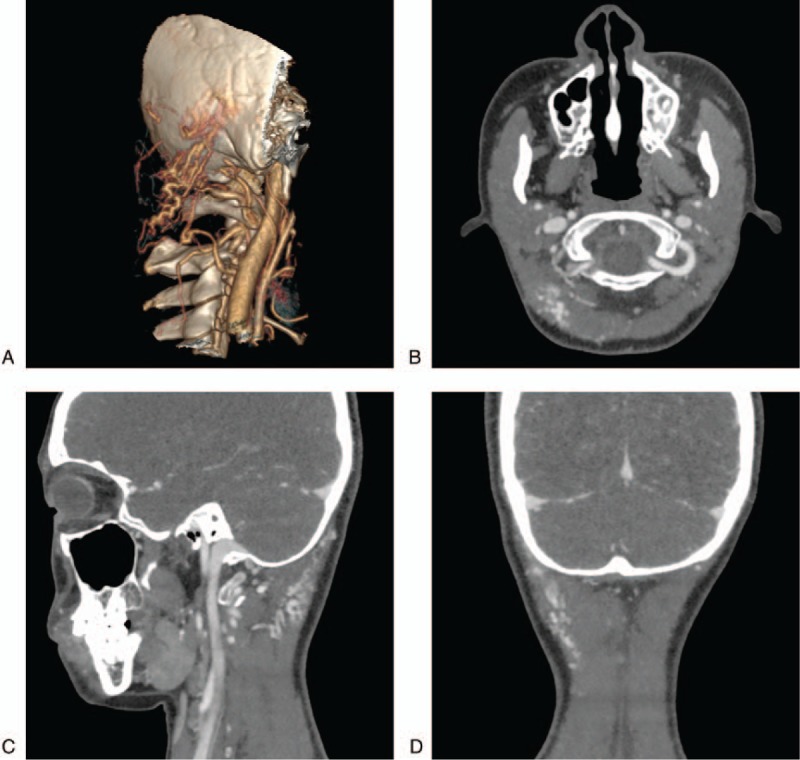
Postoperative review of CTA. A: Reconstructed CTA showing a small amount of residual lesions. B–D: Enhanced CT showing residual intramuscular hemangioma enhancement. CTA = computered tomography angiography.
